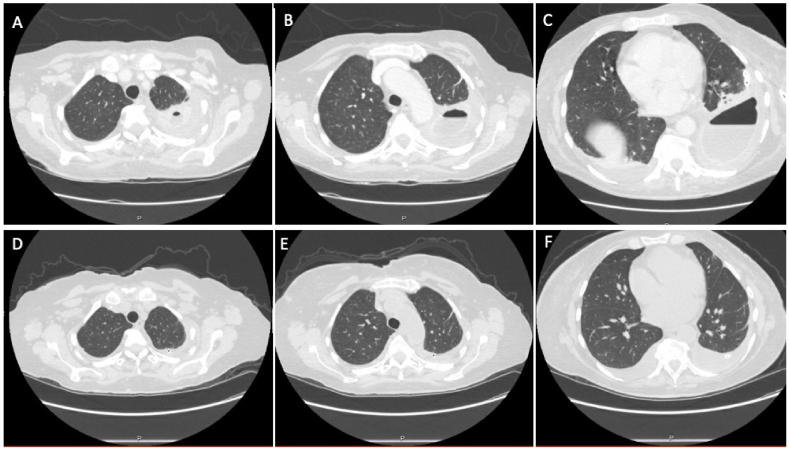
A-C Initial chest CT scan upon referral to our clinic, demonstrating intrapleural fluid collection with air-fluid level and pleural thickening. D-F Chest CT scan four-months after TPC placement demonstrating dramatic reduction in pleural collection.
